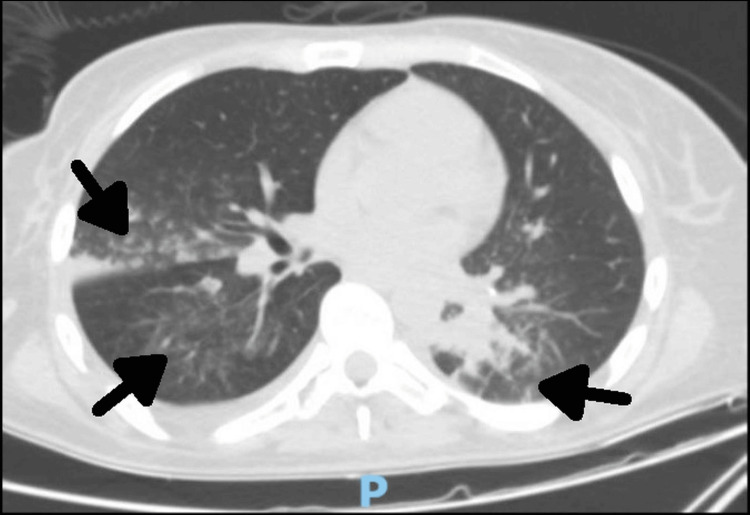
Computed tomography scan of the thorax showing ground-glass opacities (arrows) with superimposed interlobular septal thickening and intralobular septal thickening displaying the crazy paving appearance.
